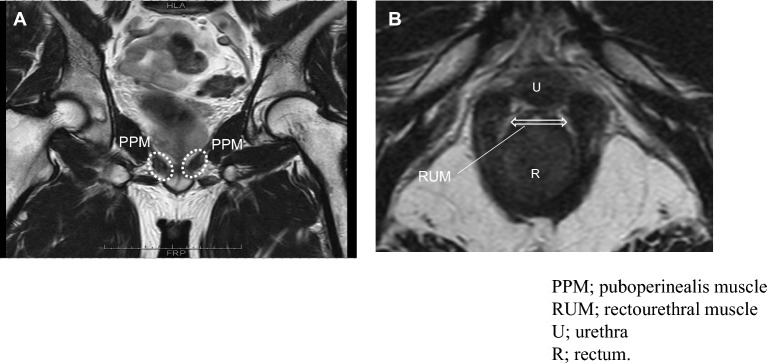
Anatomical analysis of the structure around the urethra using MRI before and after RARP. (A) The left and right cross-sectional areas of PPM (dotted circles) at the height of RUM on the dorsal side of the urethra were calculated using coronal sections. (B) The tissue between the urethra and rectum was considered to be RUM (double arrow) in the transverse plane, and the left–right diameter was measured. *PPM* puboperinealis muscle, *RUM* rectourethral muscle, *U* urethra, *R* rectum.
